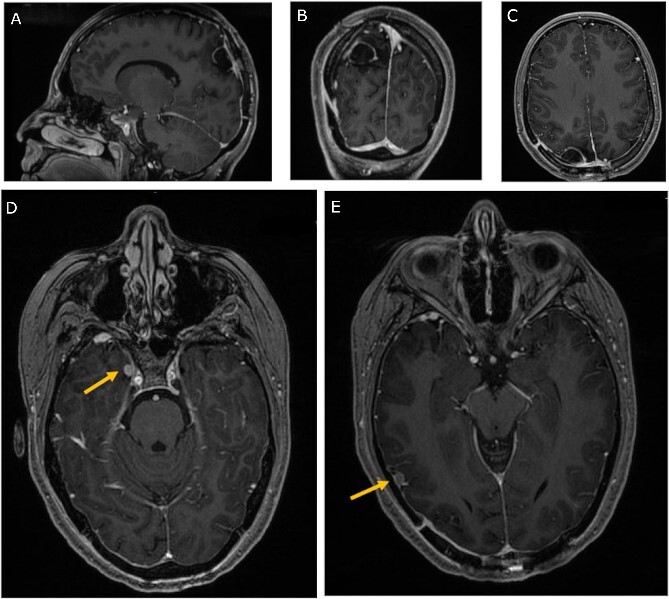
Three-month follow-up MRI; (A) sagittal, (B) coronal, and (C) axial post-contrast T1-weighted MRI scans show peripheral contrast enhancement at the parietal resection margin with areas of nodularity related to postoperative changes without recurrence; (D, E) axial post-contrast T1-weighted MRI shows stability of the medial and lateral temporal lobe lesions.
